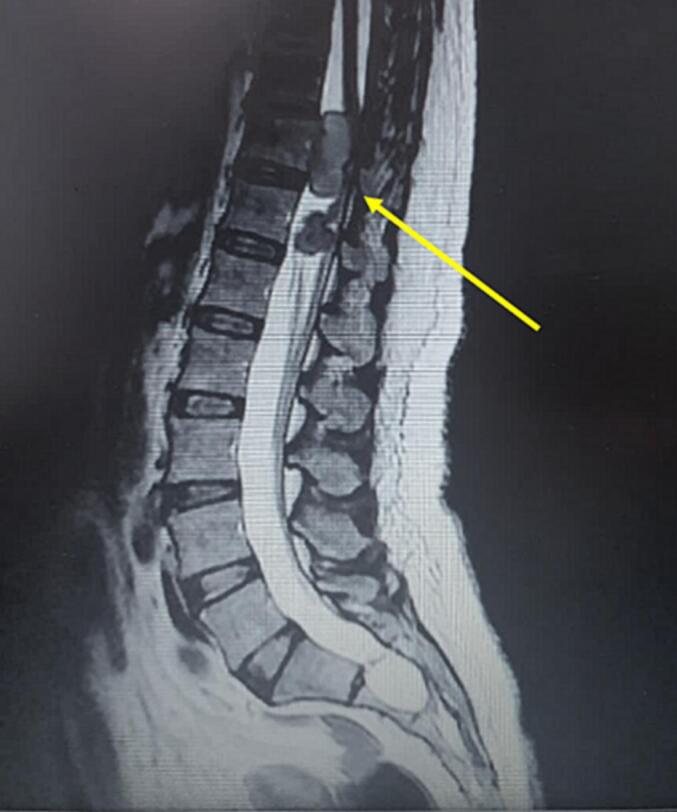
MRI Sagittal section showed multiple intramedullary tumours at the thoracal 11th and 12th vertebral levels. It measured 30 mm × 20 mm and 20mmx15mm.
